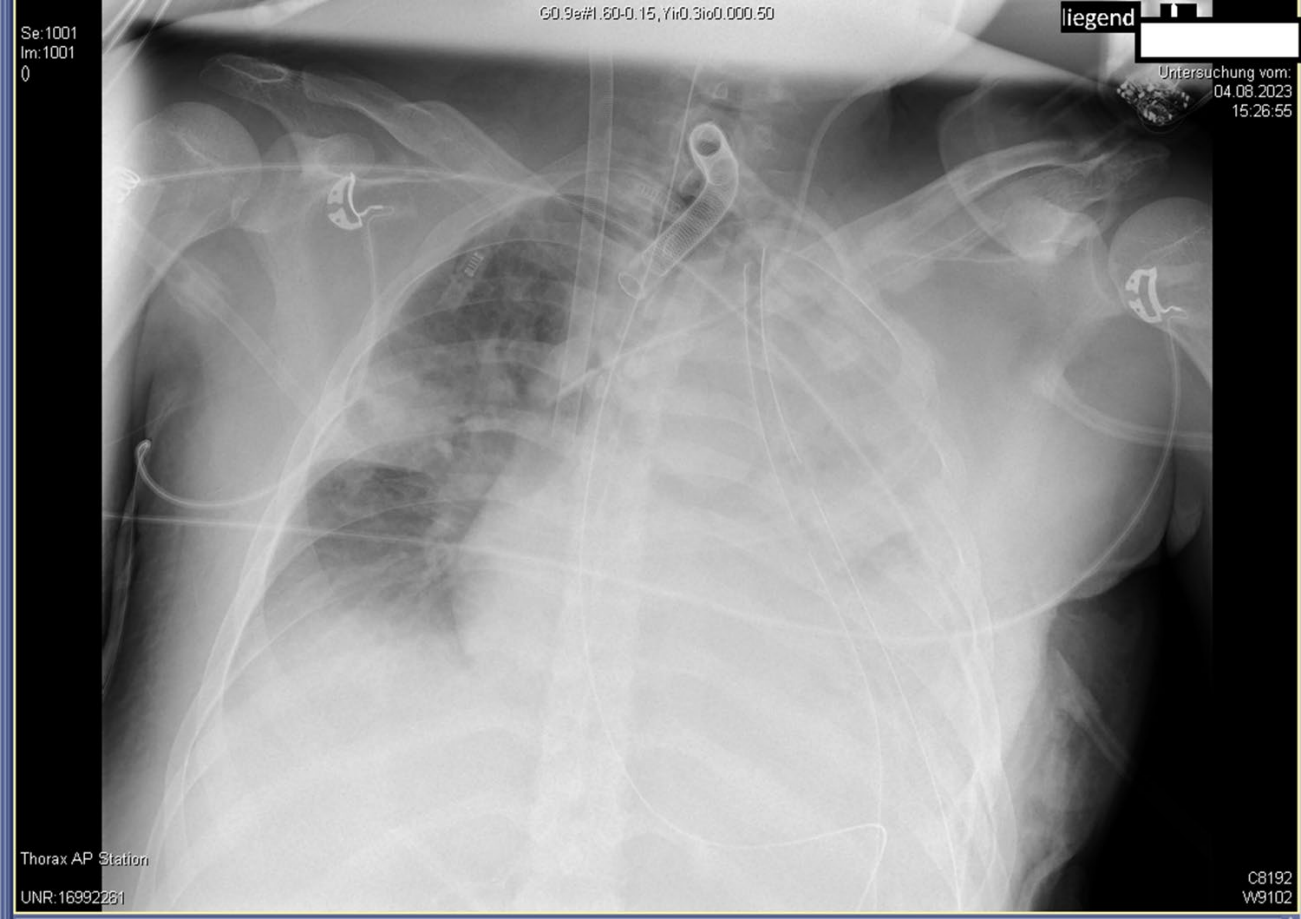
Chest radiography following ECMO and Tracheostomy tube placement in ARDS
